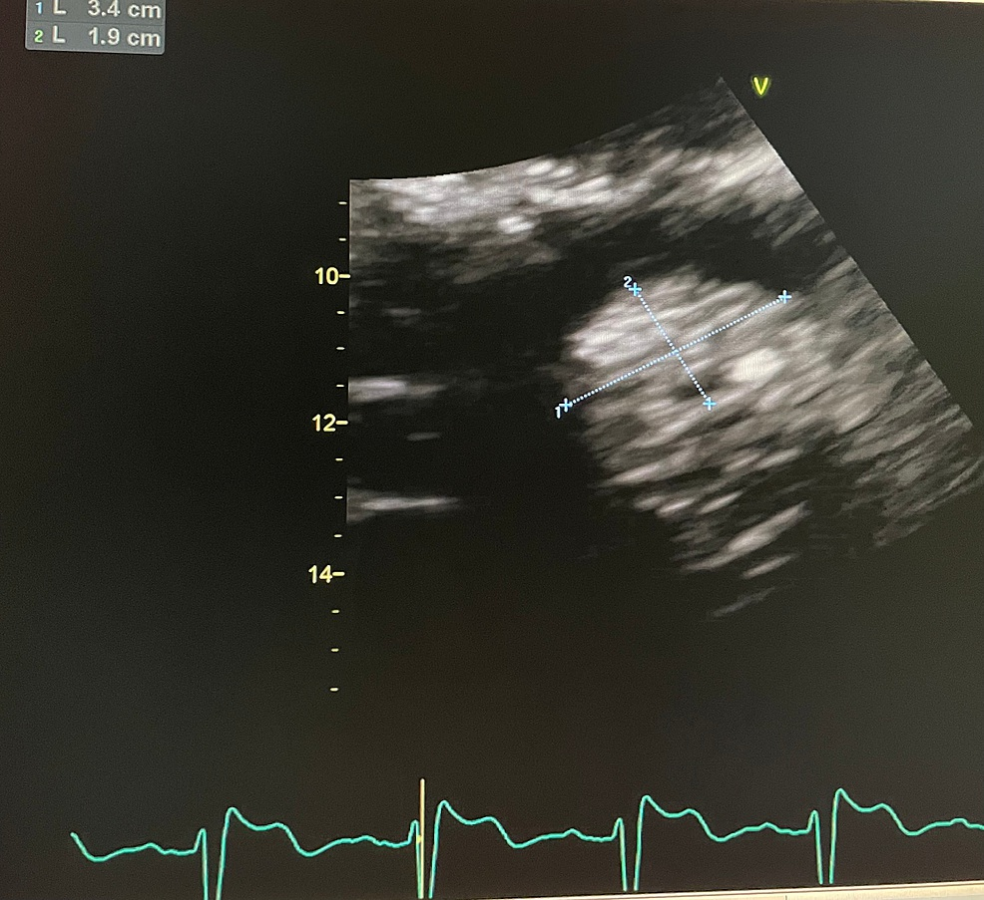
Suspected myxoma revealed by TTE TTE: Transthoracic echocardiogram
